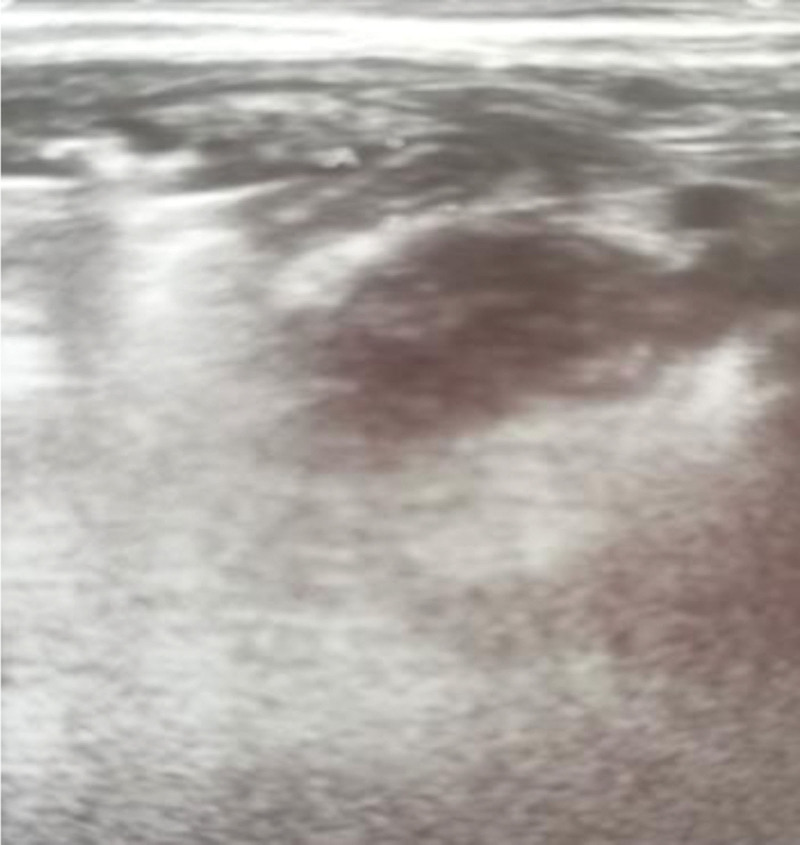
Abdomen ultrasound in patient #1 showing inflamed appendix.
